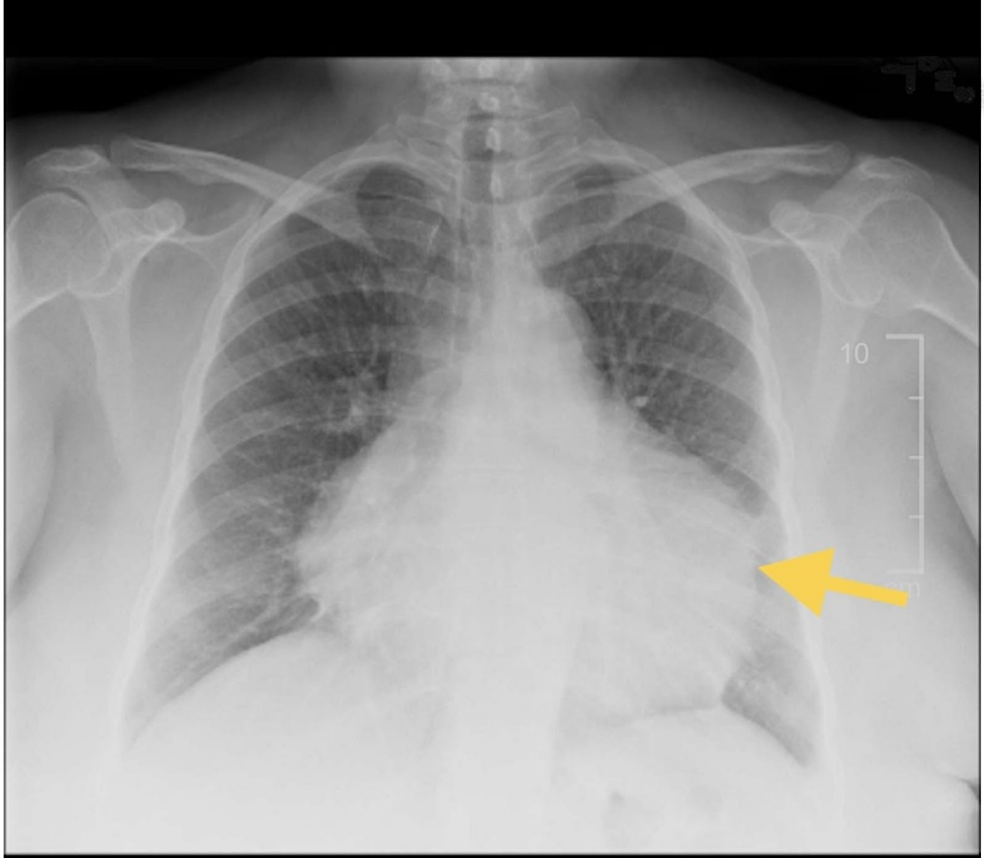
Chest x-ray anterior-posterior (AP)/posterior-anterior (PA) with the yellow arrow showing an enlarged cardiac silhouette
